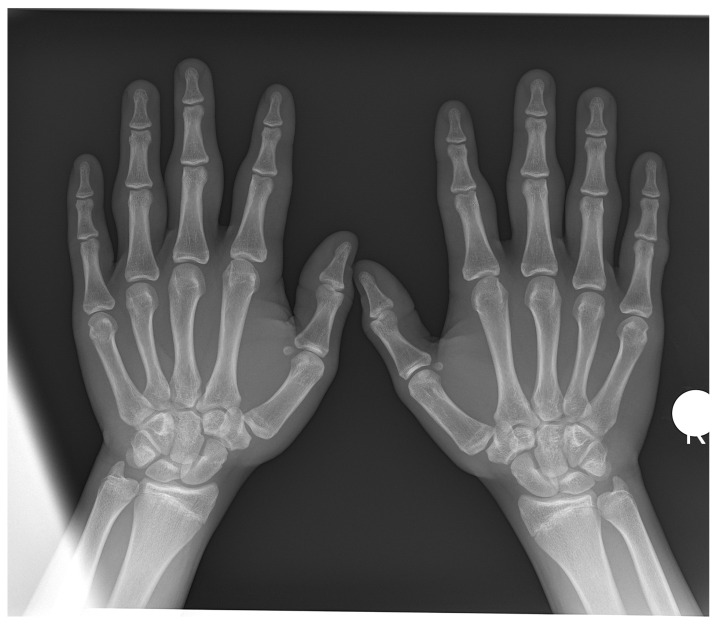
Plain radiograph of both hands (Case 2)—normal bone and joint structure, with no osseous erosions, articular space narrowing, or periosteal changes.
